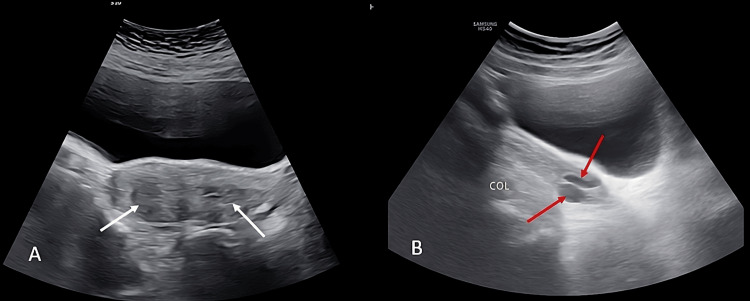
Suprapubic ultrasound showing two non-divergent uterine cavities (A: white arrow) and two cervical canals (B: red arrow)
